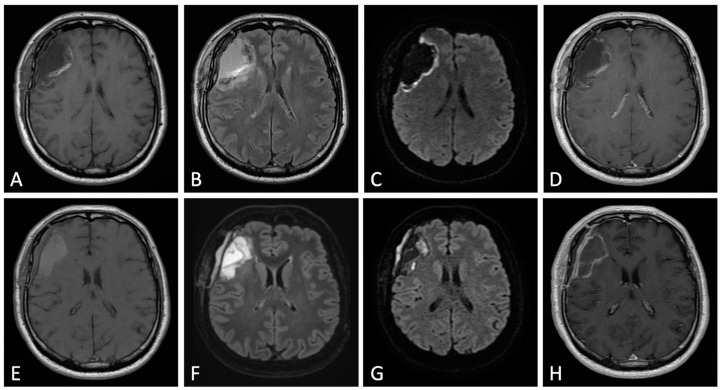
Differences between early (48 h, upper row) and delayed (one week, lower row) post-operative MRI. Axial pre-contrast T1w (A,E), FLAIR (B,F), DWI (C,G), and post-contrast T1w (D,H). One week after surgery, pre-contrast T1w image (E) shows marked T1 shortening caused by the formation of methemoglobin within the surgical bed. “Benign” widespread enhancement also occurs along the resection margins (H); it does not allow for the distinction of residual tumor from surgically induced enhancement.
